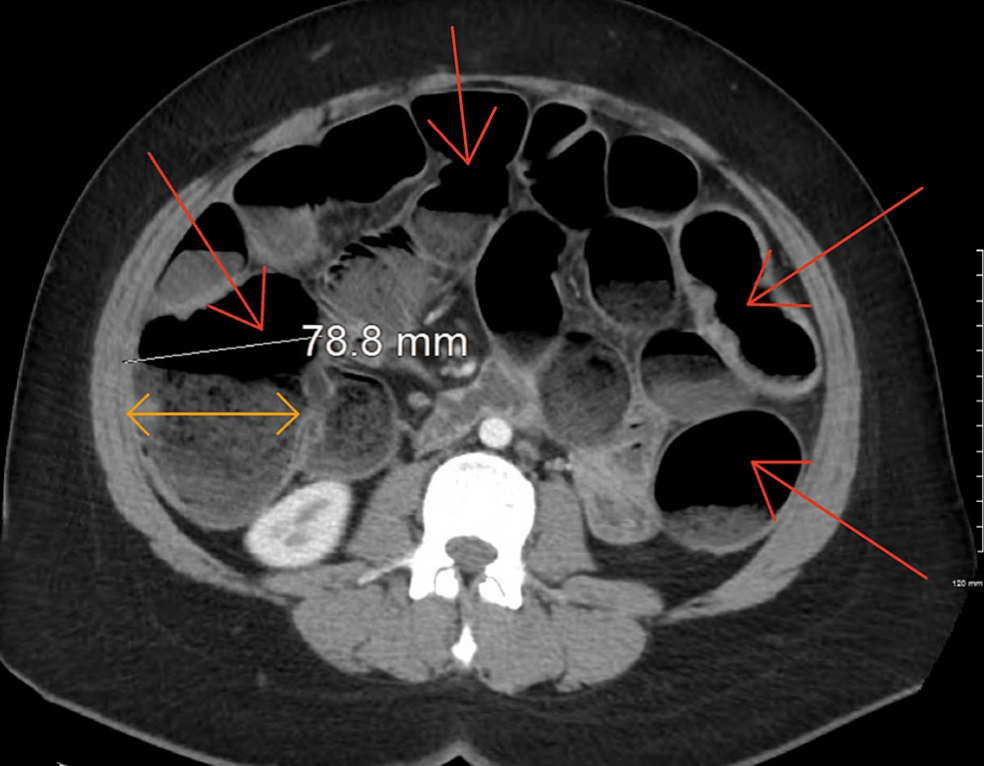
Axial CT scan. Red arrows show severe distention of the small and large bowels. The orange arrow shows distention of the colon, with the cecum measuring nearly 8 cm in diameter. CT, computed tomography
